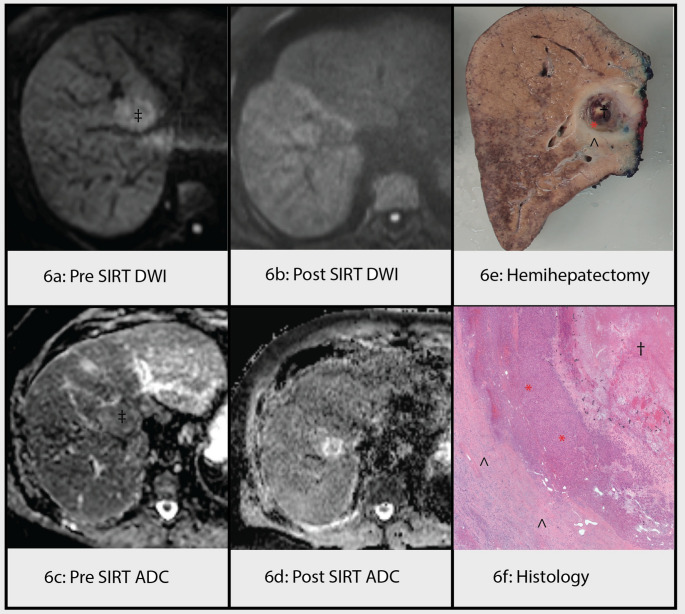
a–f Tumour (‡) demonstrating persistence of diffusion restriction on DWI and ADC map post SIRT (a–d; no arterial phase was available so mRECIST could not be assessed). Residual viable tumour (*) was present at the periphery (estimated 50%) of tumour with a necrotic core (†), and surrounding fibrosis (^)
